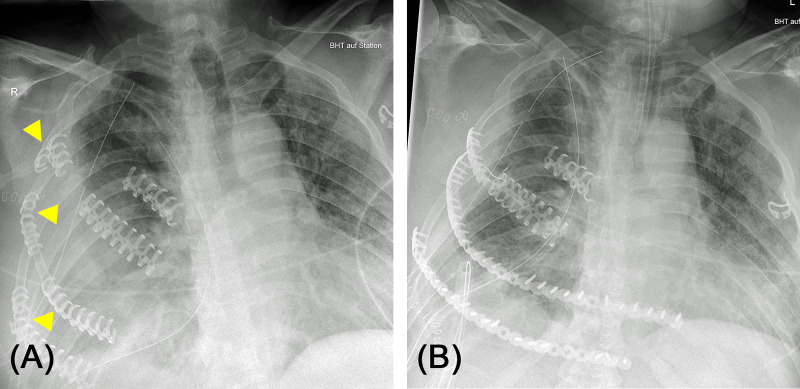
(A) 63-year-old male patient after car accident. Surgery for thoracic trauma was carried out at the day of hospital admission including rib fixation. The patient suffered from severe delirium afterwards. At day 7, chest X-ray revealed several broken osteosynthetic clips (arrows) causing recurrent instability of chest wall. (B) Post-operative chest X-ray after re-stabilization of the chest wall replacing broken clips with screwed titanium plates.
